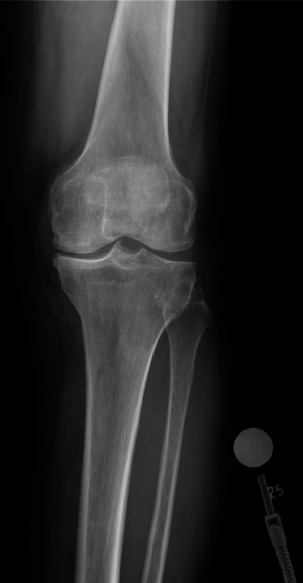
Preop anterior knee X-ray.
